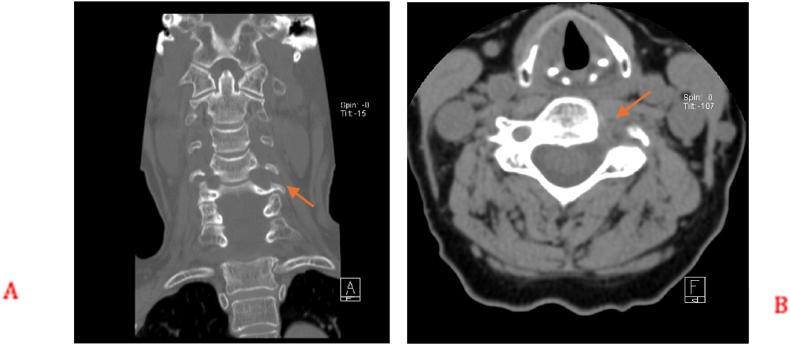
(A) CT spine coronal view showing enlargement of the left C4-5 foramen. (B) Axial CT scan through C4-5 Foramen.
